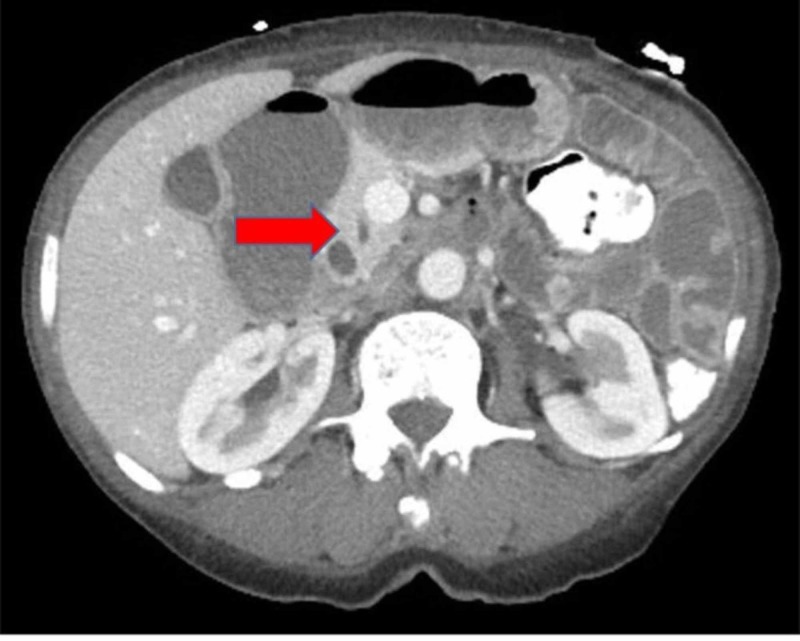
A computed tomography (CT) image with contrast demonstrates a gastric outlet obstruction, along with tapering of the third portion of the duodenum
